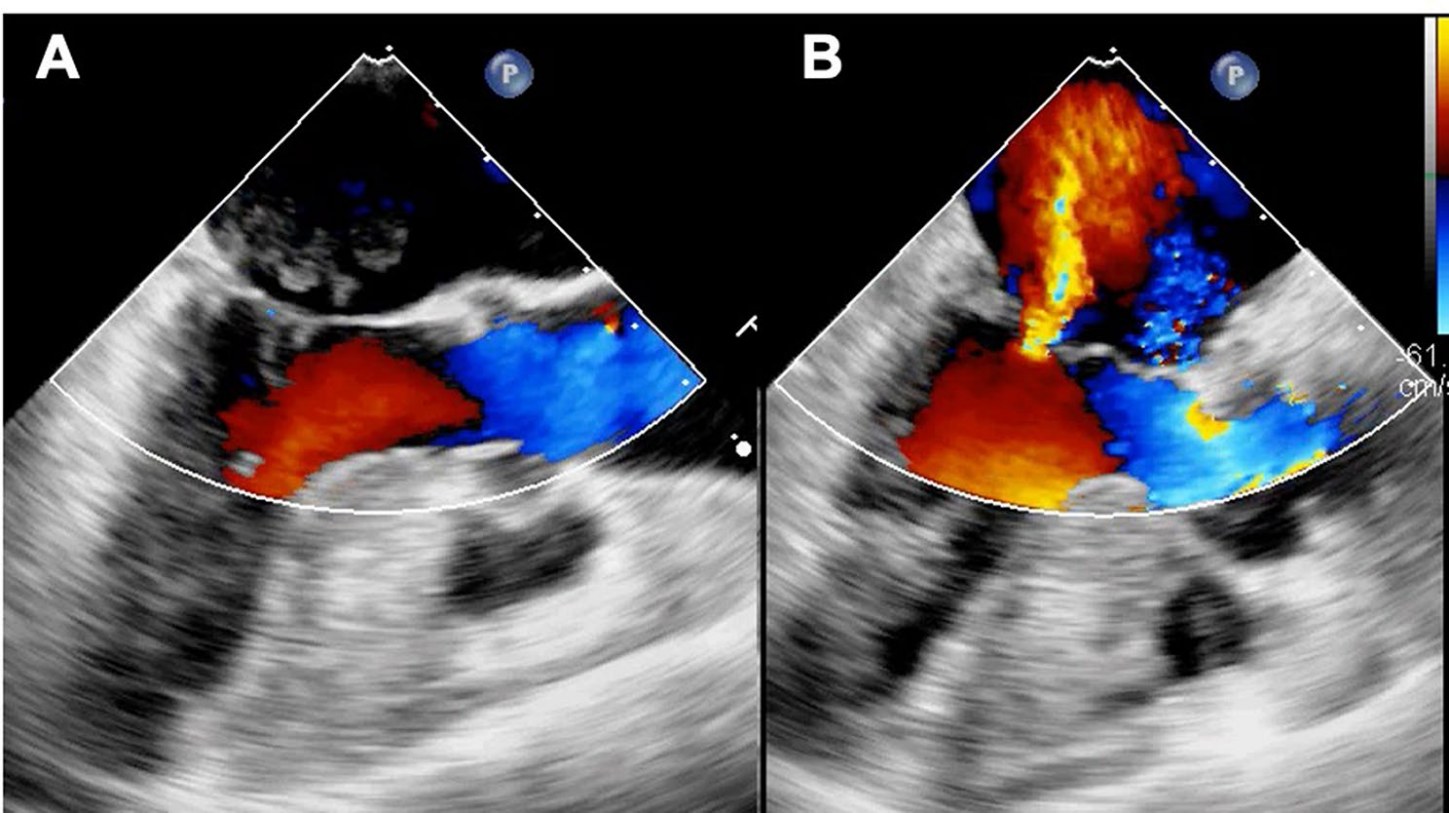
Intraoperative transesophageal echocardiography. Before tumor resection, no significant MR was detected (A). After tumor resection, severe MR due to A2 prolapse was demonstrated for the first time (B). MR: mitral regurgitation
